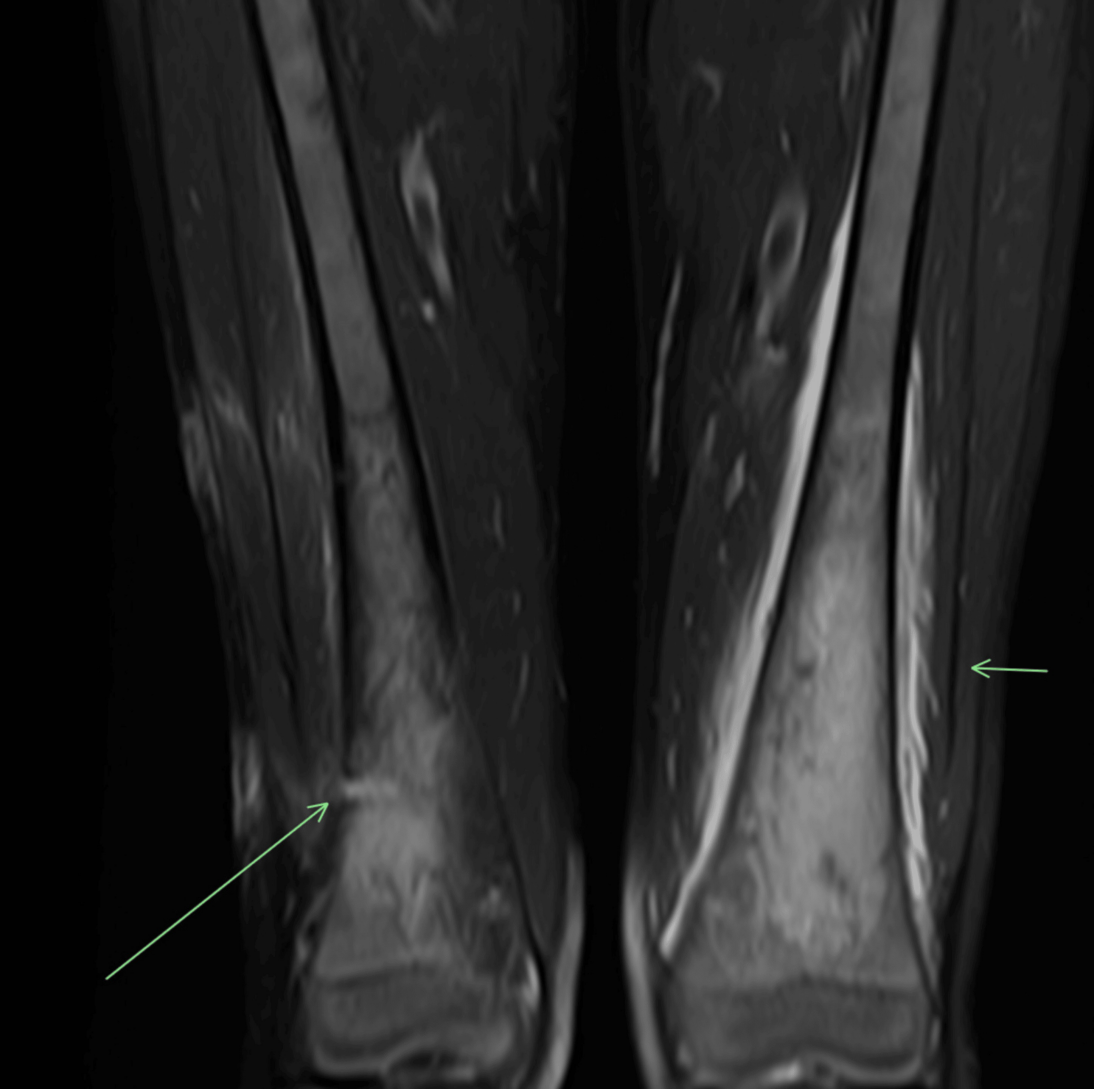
The 1.5T MRI coronal fat-saturated T2-weighted image of the bilateral femora Extensive marrow edema is noted in the left femoral metadiaphysis with subperiosteal fluid and paraosseous myositis (short horizontal arrow). Residual finding of marrow edema is observed in the right femoral metaphysis, with a lateral biopsy tract (long oblique arrow) and no significant extraosseous findings.
